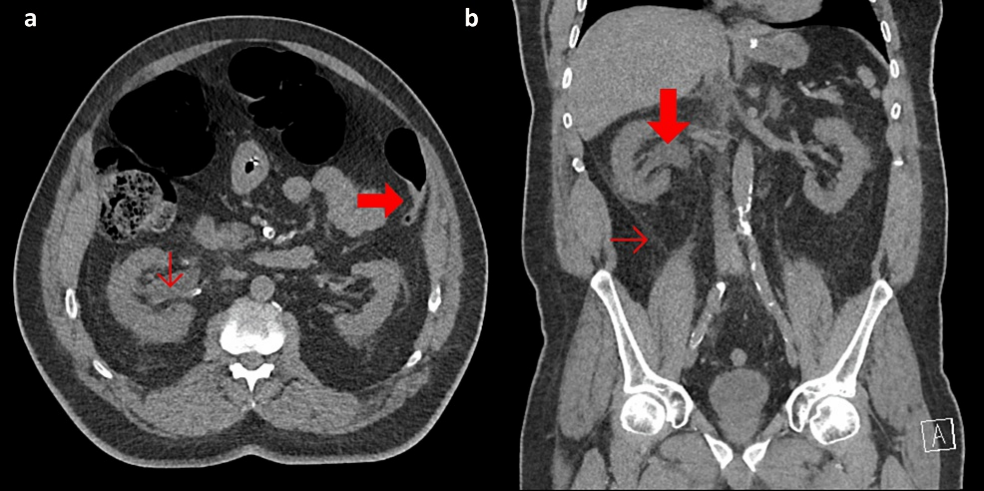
Non-contrast computed tomography scans on admission. The image show (a) axial slice demonstrating right hydroureter with surrounding perinephric stranding (thin red arrow) and re-demonstration of the abrupt transition at the splenic flexure (thick red arrow), and (b) coronal slice demonstrating right hydroureter (thick red arrow) and increased attenuation of the Gerota fascia (thin red arrow).
